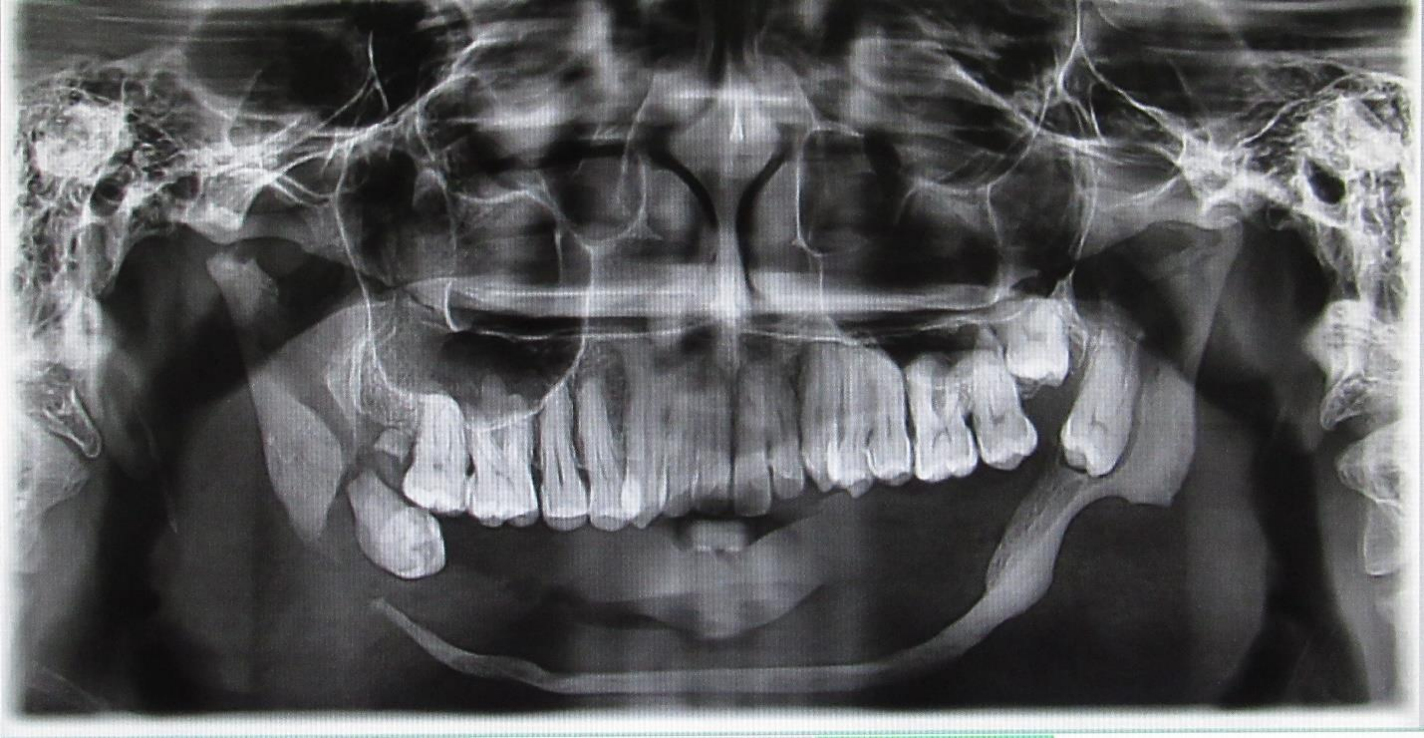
Orthopantomograph showing resorption of the mandibular arch and pathological fracture in the right lower border of mandible.
